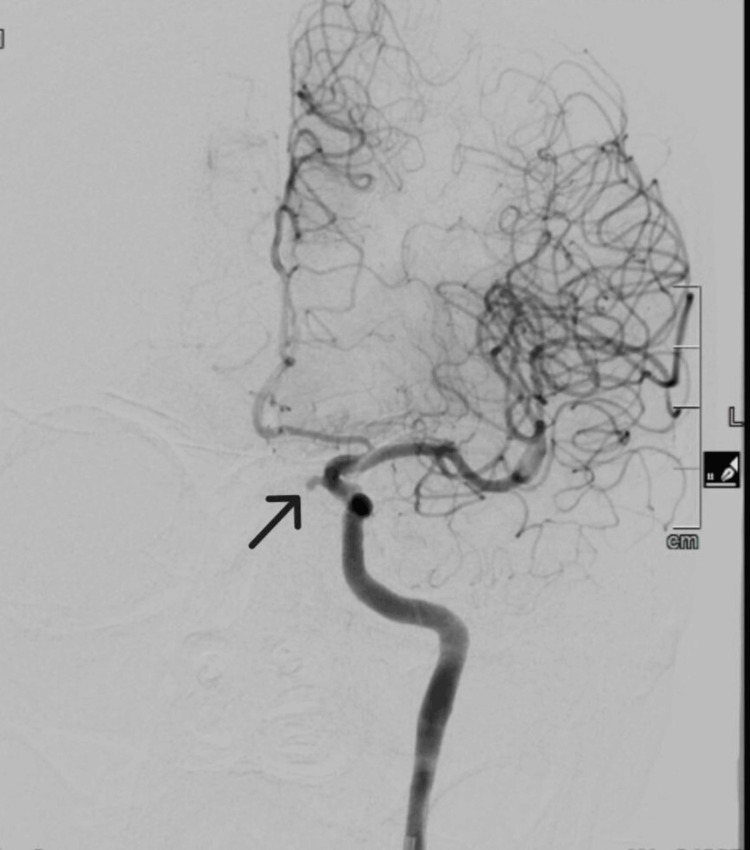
Coronal CT angiography before coiling, demonstrating a supraclinoid segment ICA aneurysm (arrow) ICA: internal carotid artery
